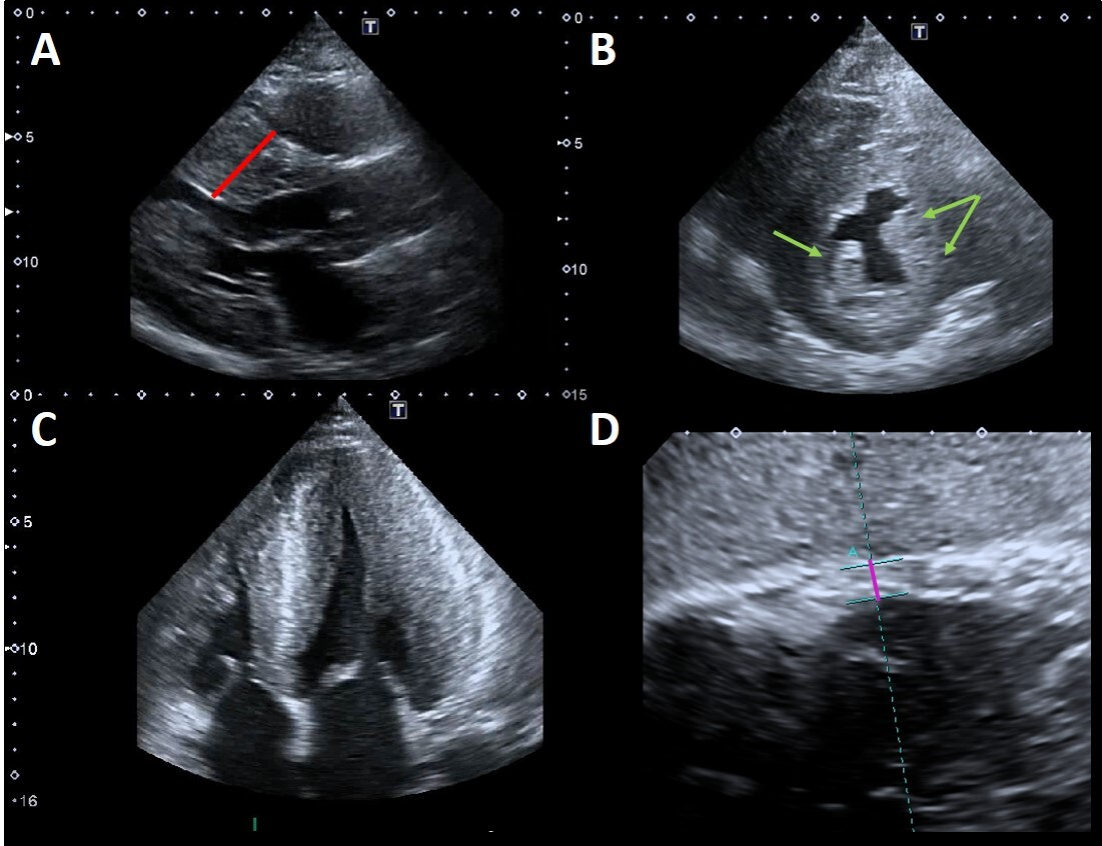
Example of advanced Fabry cardiomyopathy. (A) Parasternal
